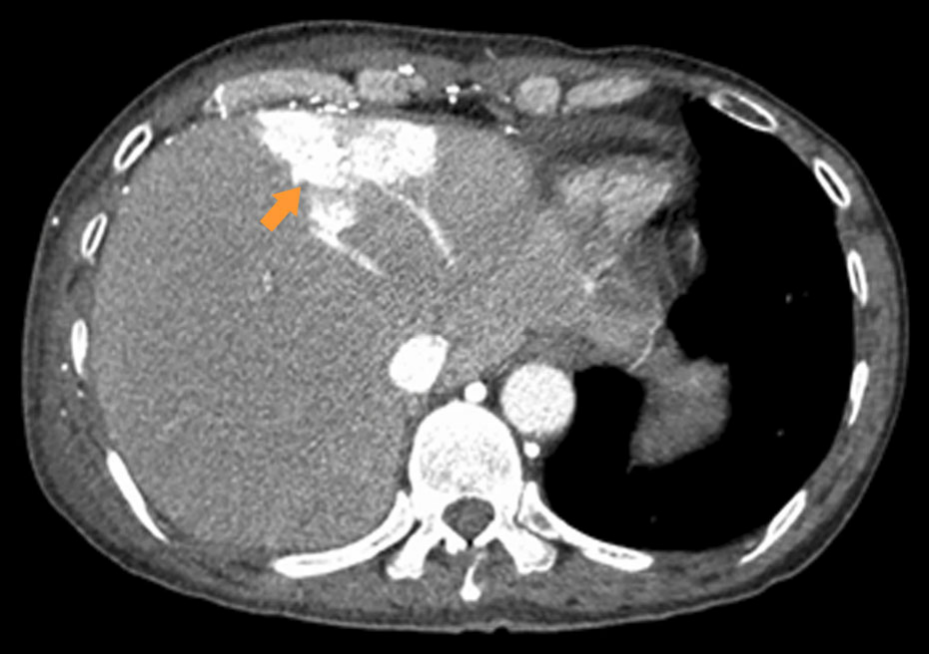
The focal hepatic hot spot sign on the axial contrast‐enhanced computed tomography (CT) image in the arterial phase. It manifests as an intense wedge‐shaped homogeneous enhancement area of size 4 × 6 cm in segment IV of the liver (arrow).
